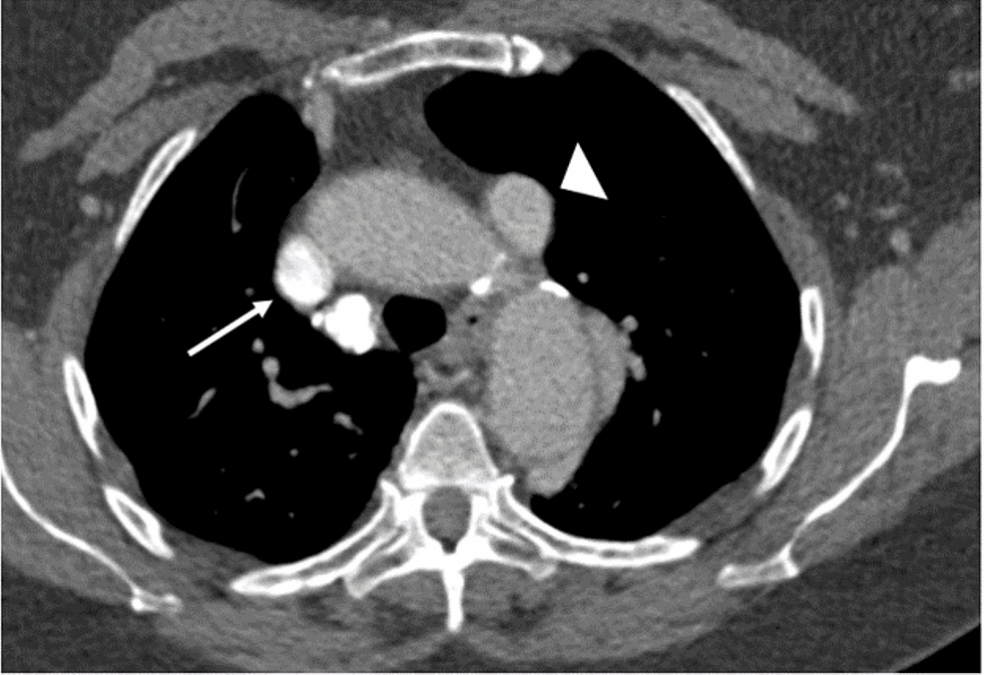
Axial CT image from the initial CT angiogram of the chest injected through the right upper extremity. The right (white arrow) and left (white arrowhead) SVC are both seen on this slice, with the more dense contrast bolus seen in the right SVC. SVC: superior vena cava.
